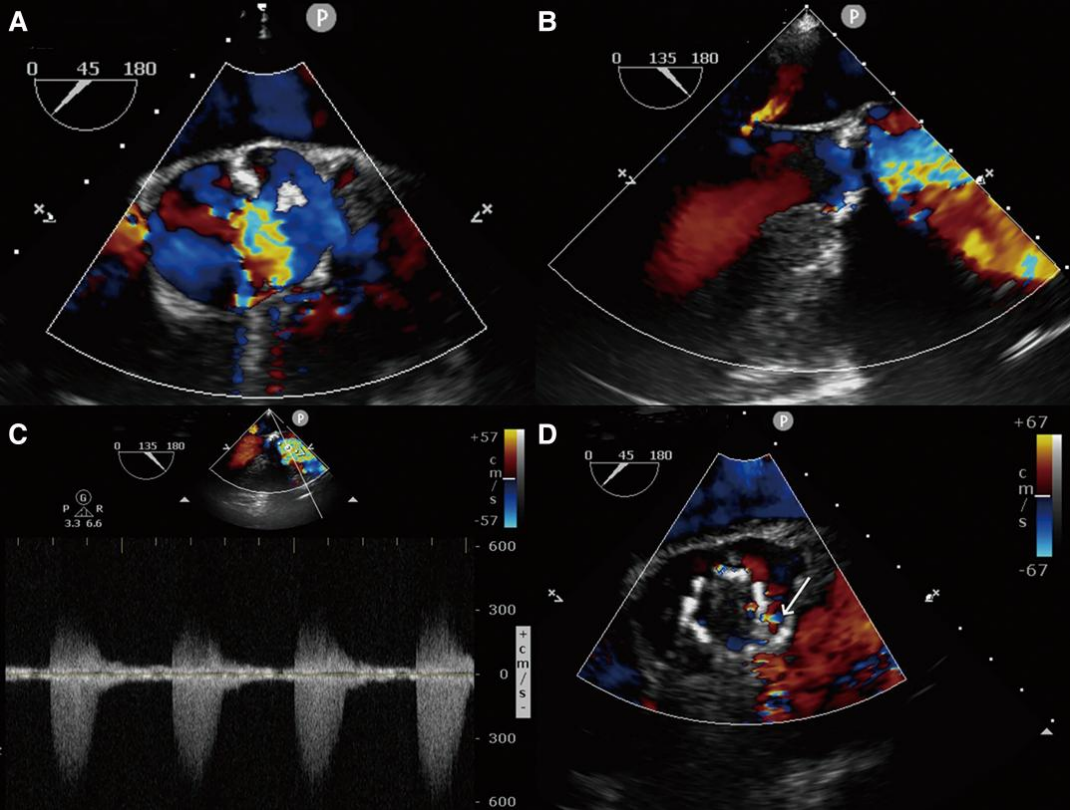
Pre-operative and post-operative echocardiography. (*A–C*) Pre-operative echocardiography: severe aortic stenosis; (*D*): Post-operative echocardiography: slight paravalvular leakage (white arrow).
